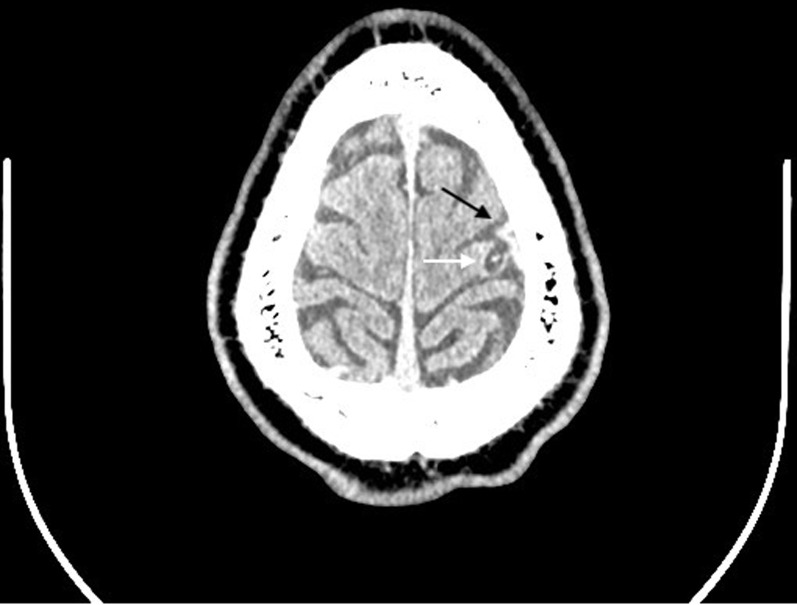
contrast-enhanced axial computed tomography brain showing a developmental venous anomaly (black arrow) adjacent to a cystic lesion in the posterior frontal lobe without surrounding oedema (white arrow)
